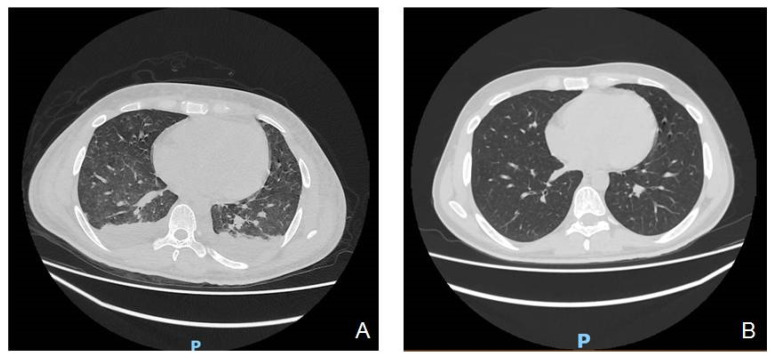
(A,B) CT scans of progressive improvement and disappearance of pulmonary fungal involvement were evaluated after 7 (A) and 20 days (B) from the start of antimycotic therapy. P, posterior of the chest.
